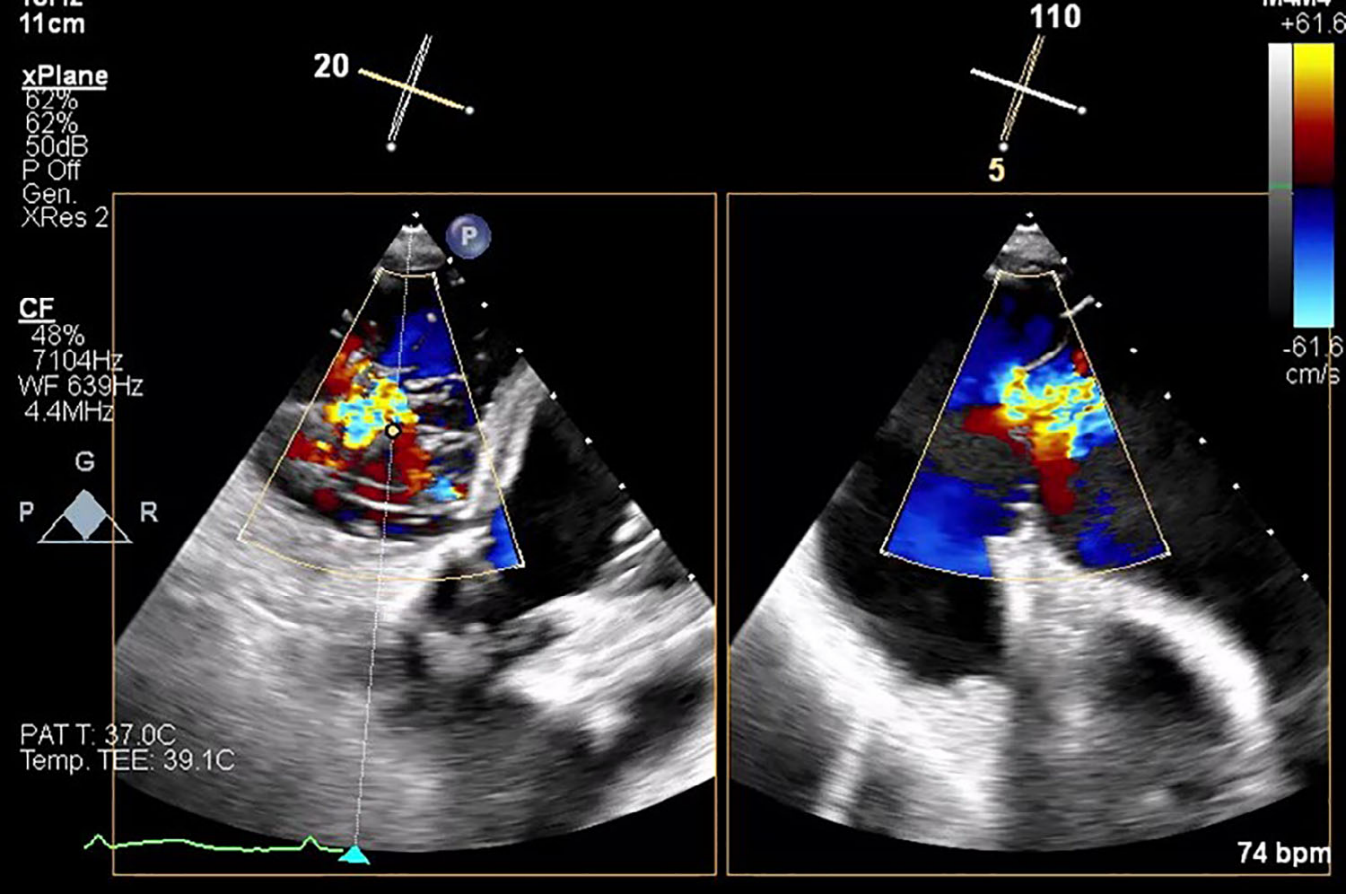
TOE TG view color Doppler and simultaneous biplane imaging that shows a “central” regurgitation (see GLIDE score). TG indicates trans gastric; TOE indicates transesophageal echocardiography.
